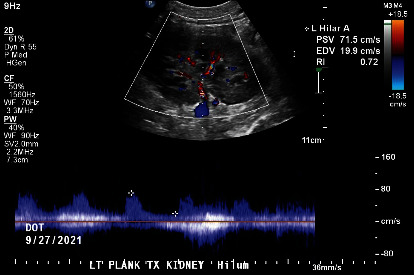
Normal echogenicity and resistive indices of the transplanted kidney as shown by Doppler US.
